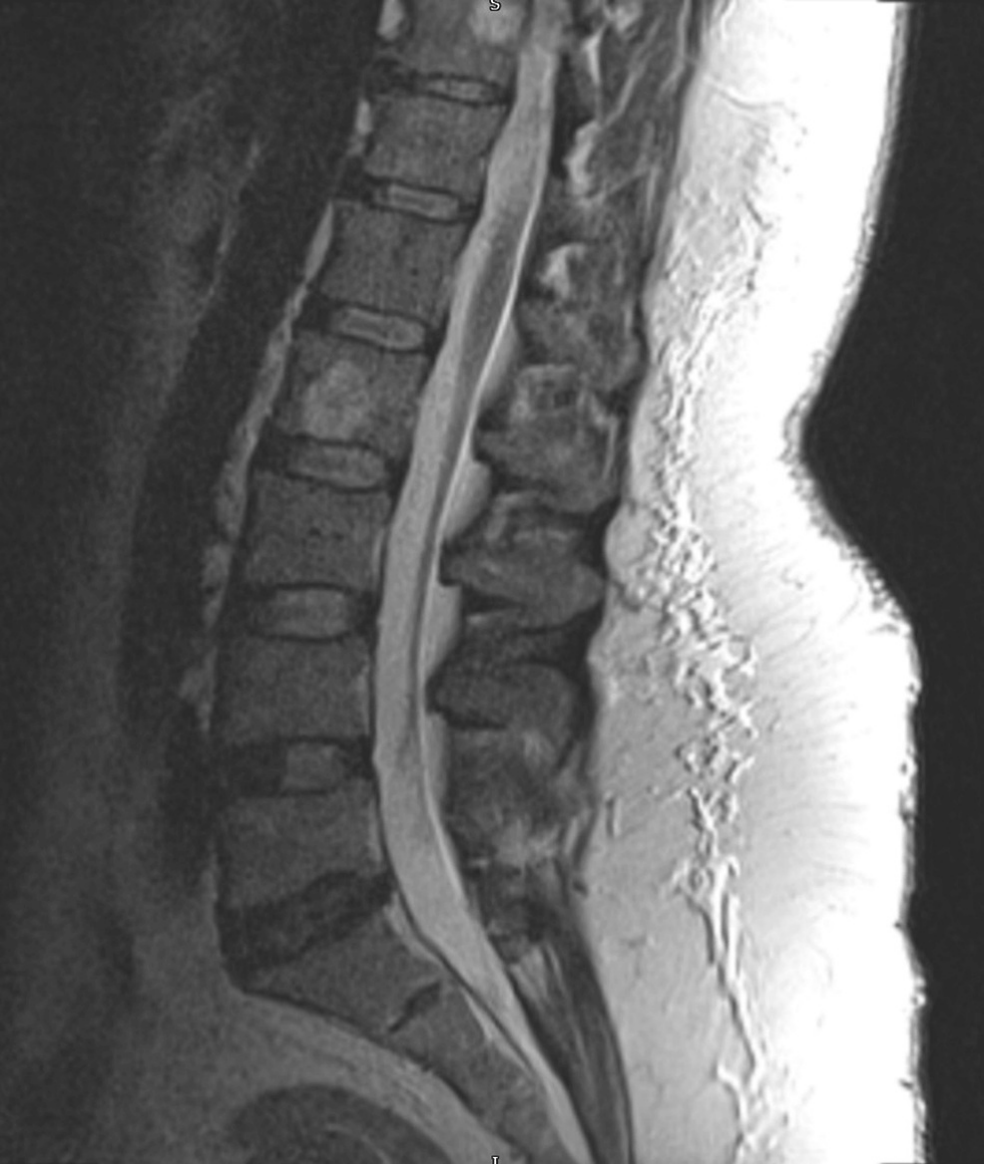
T2W sagittal MRI LS spine showing normal curvature, mild disco-vertebral degeneration, and incidental hemangiomas LS spine, lumbosacral spine; T2W, T2-weighted
